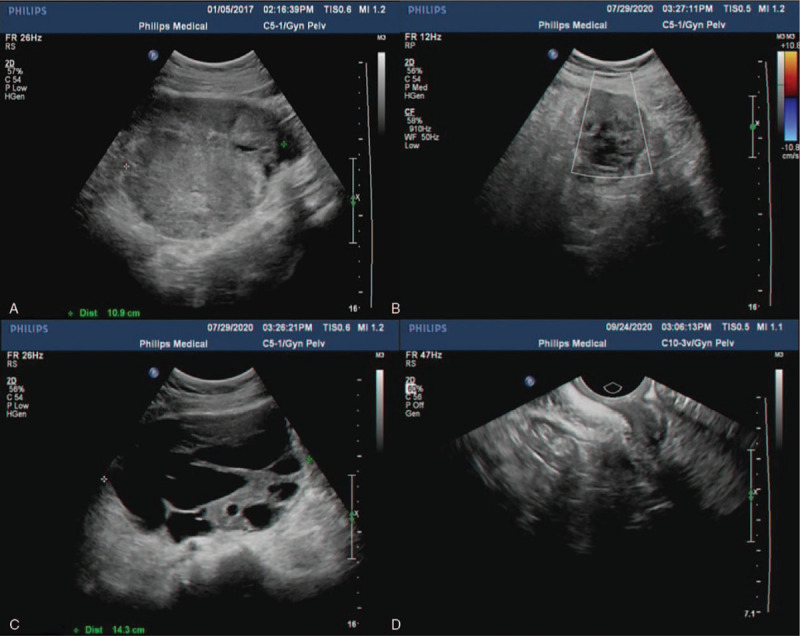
Gynecological ultrasound of Case 2. (A, B, and D) Ultrasound revealed a 6.3 cm × 5.1 cm heterogeneous cystic mass with an irregular shape, unclear boundary, and no blood flow. (C) Above the vaginal stump, we found a 14.3 cm × 8.0 cm cystic mass with regular morphology, no internal blood flow, and multiple compartments.
